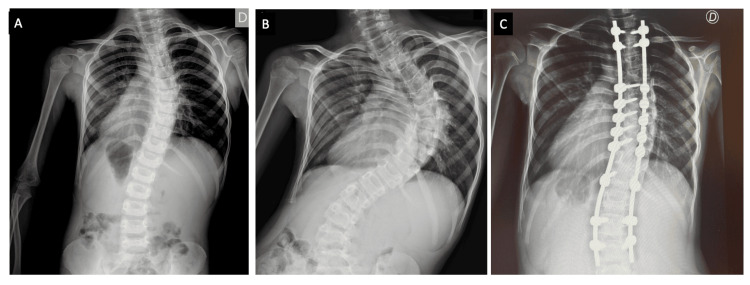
Patient scoliosis radiographs demonstrate the progression of scoliosis over time. A) Scoliosis at 5 years of age. B) Severe thoracolumbar scoliosis at 10 years of age, with a Cobb angle of 45 degrees. C) Post-surgical radiograph showing correction of the deformity.
